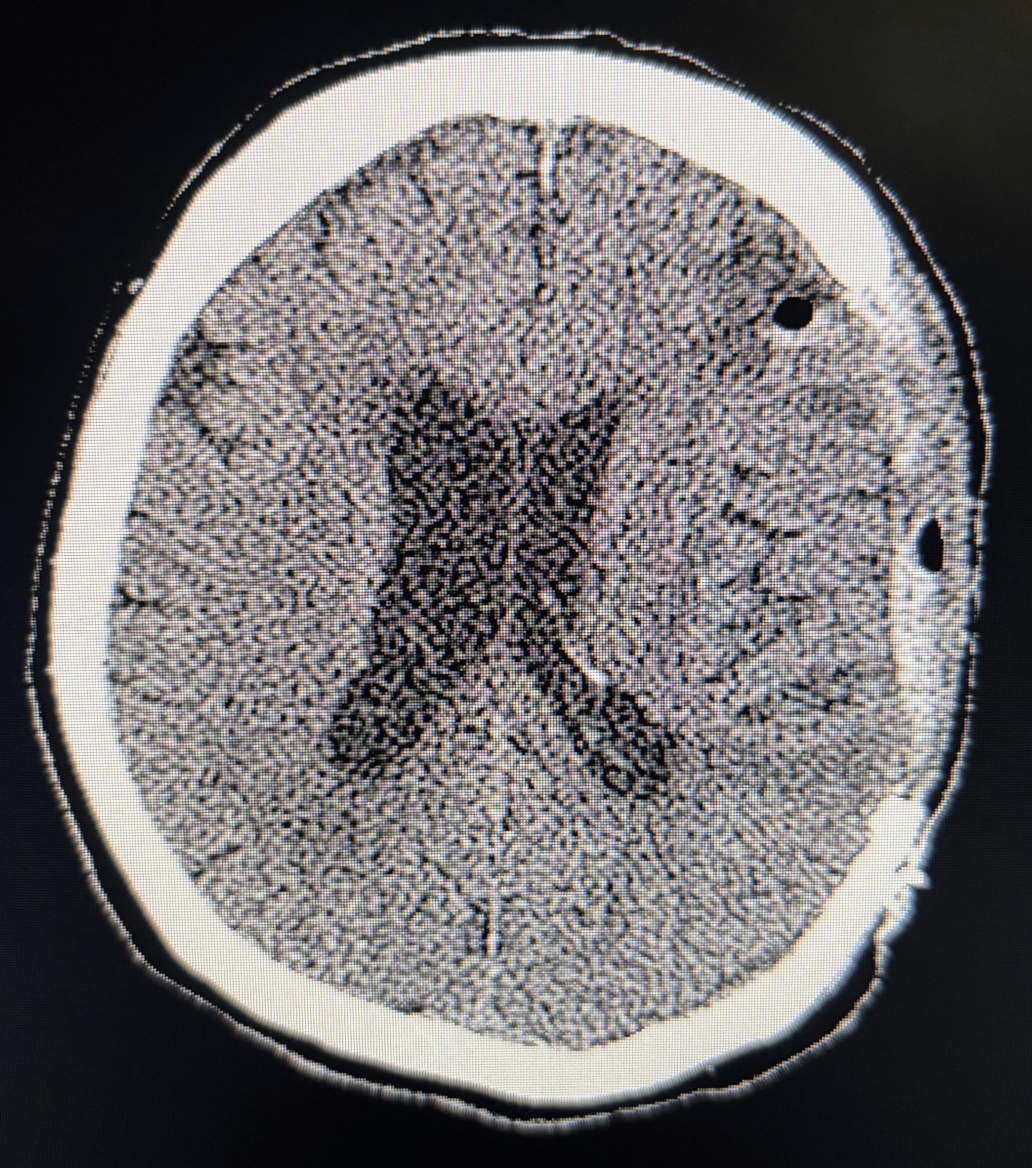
Resolution of Midline Shift CT imaging shows complete resolution of midline shift after NegPresHelm intervention.
